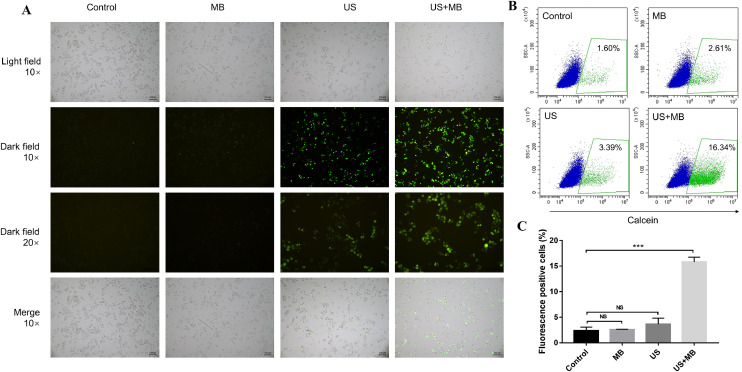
US-mediated MB cavitation increased cell permeability. (A) Fluorescence images of calcein-postive cells. (B and C) Flow cytometry results of calcein-positive cells. US, ultrasound; MB, microbubbles; * *P* < 0.001; NS: non-significant.
